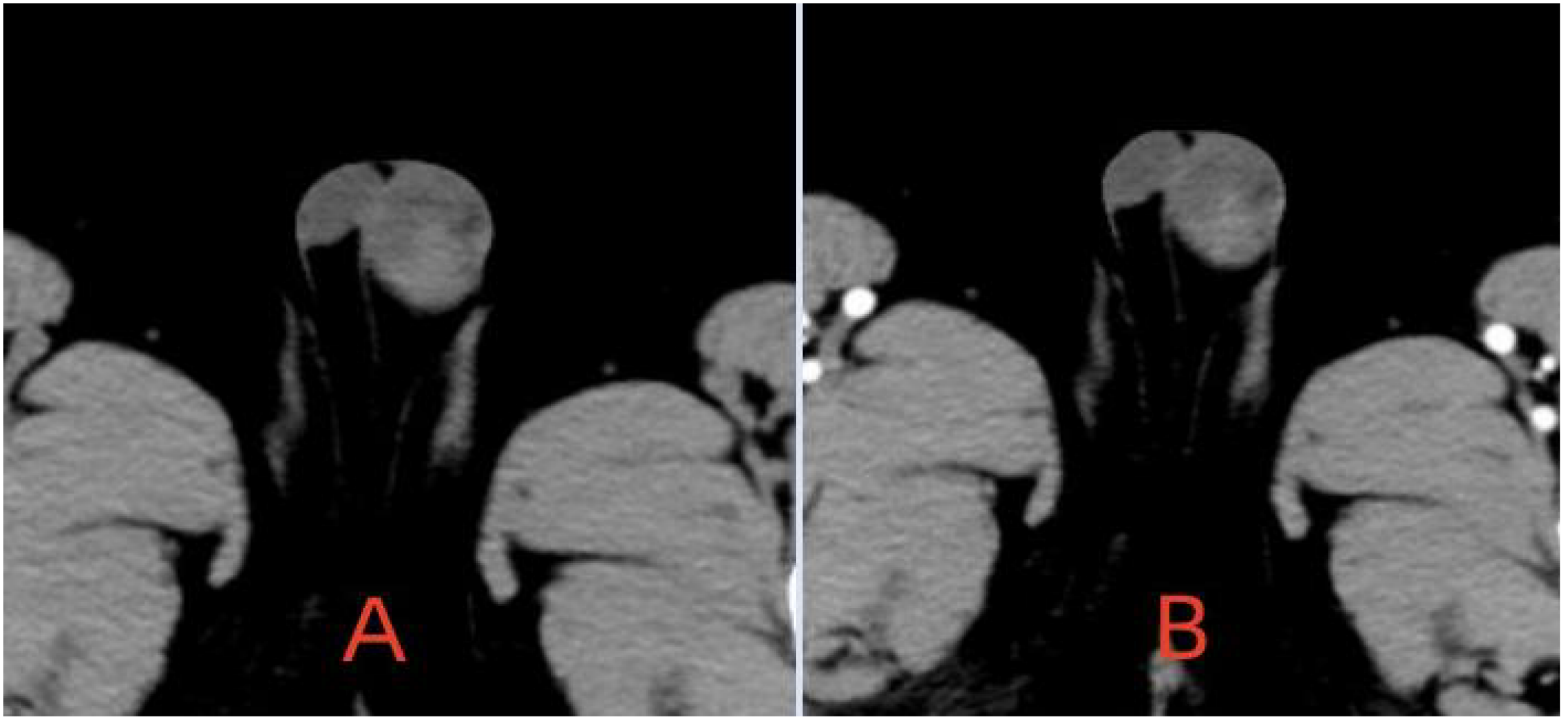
(A) CT scan. (B) CT enhancement showing the enhancement of some testicular tissue.
